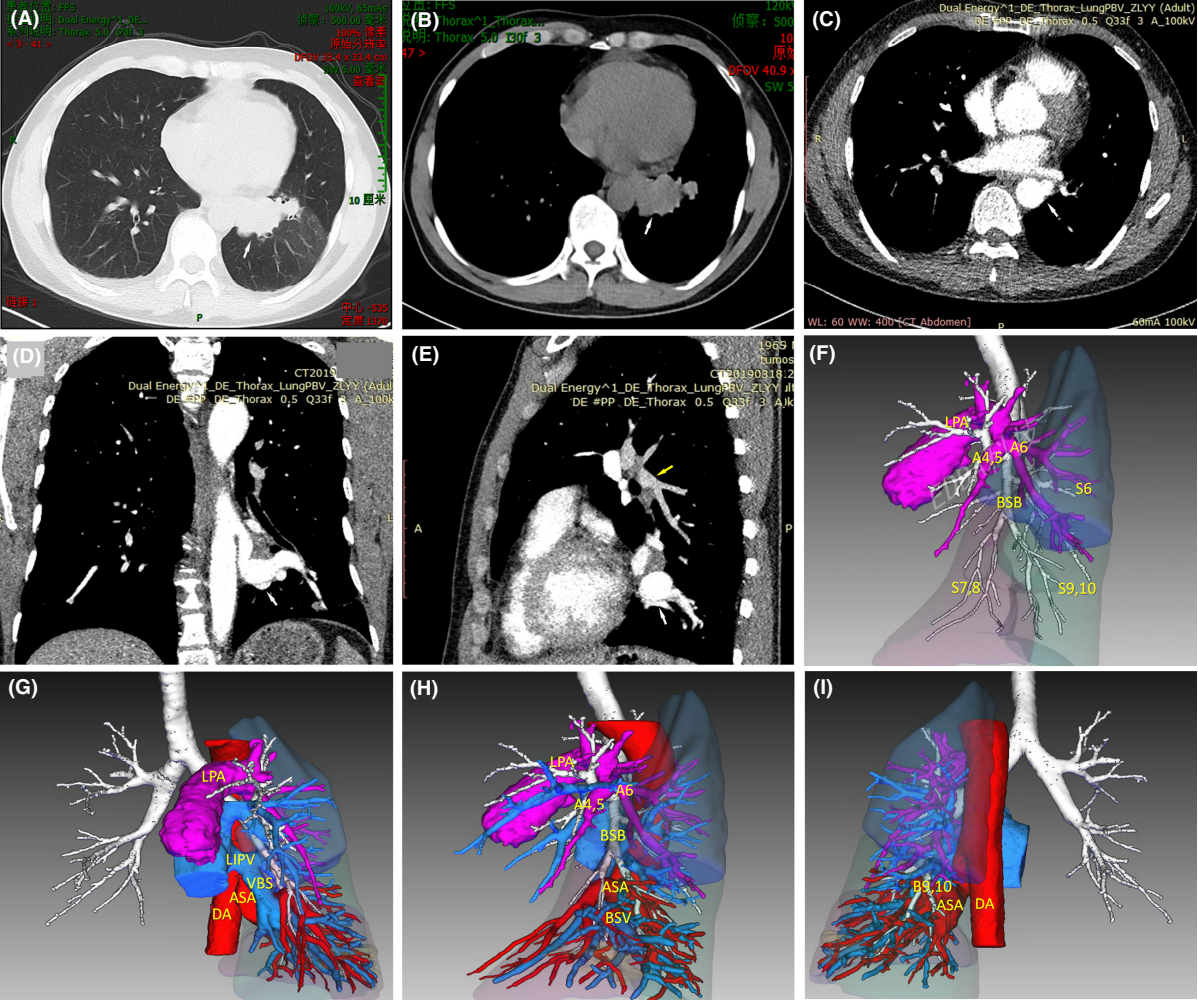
Computed tomography (CT)/CT angiography and three‐dimensional (3D) reconstruction depicting the anomalous systemic arterial (ASA) supply to the normal basal segments of the lung (A). (B) A plain CT showing a mass in the left lower lobe. Contrast‐enhanced CT angiography showing the origin and supply of the ASA (white arrow) in coronal (C), axial (D) and sagittal (E) views. The left pulmonary artery (PA) ended in the dorsal segment (yellow arrow). (F–I) Merged three‐dimensional reconstructed image used to locate the ASA (G) and (I), which substituted the PA basal segments (F) and (H) showed that the bronchi and pulmonary vein connected normally. A4,5, lingual artery segment; A6, dorsal artery segment; ASA, anomalous systemic arterial; BSB, basal bronchial segment; B9,10, bronchi of the lateral and posterior basal segments; DA, descending aorta; LIPV, left inferior pulmonary vein; LPA, left pulmonary artery; S6, dorsal segment; S7,8 median and anterior basal segment; S9,10 lateral and posterior basal segments; and VBS, basal vein segments.
